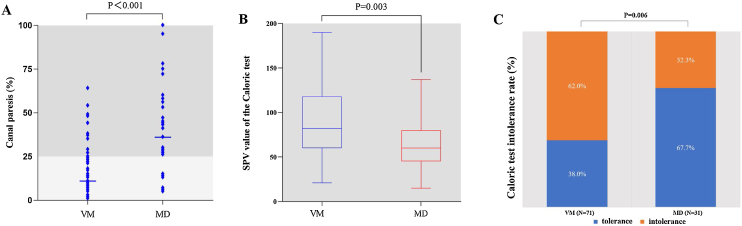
Comparison of CT results between VM patients and MD. (A) Horizontal semicircular canal function in VM and MD patients. (B) The SPV of caloric-induced nystagmus in VM and MD patients. (C) The incidence of CT intolerance in VM and MD patients.
